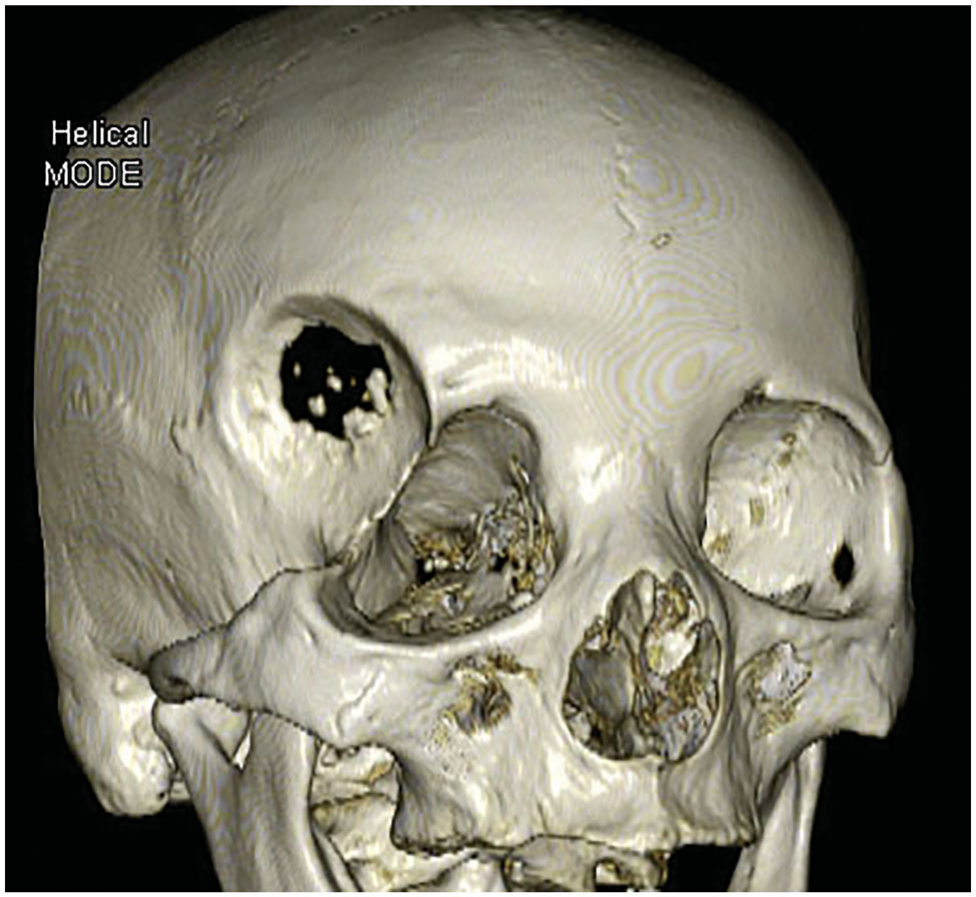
Computed tomography shows full-thickness bone erosion at the right frontosphenoidal suture and orbital disfigurement.
